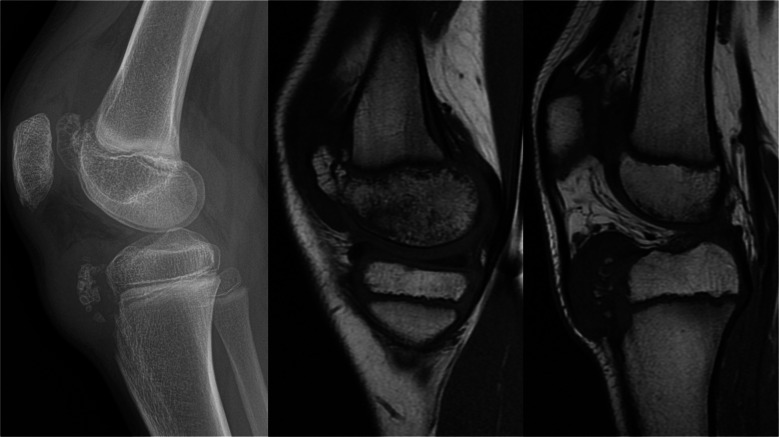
Eight-year-old female with Trevor disease. Lateral knee radiograph (right) demonstrates an ossified mass adjacent to the distal femoral epiphysis with distortion of the quadriceps insertion. Multiple discrete areas of ossification in the region of the tibial apophysis with distortion of the patellar tendon contour. T1-weighted sagittal images (centre and left) confirm marrow fat signal within the regions of ossification within surrounding low T1 un-mineralised cartilaginous components
